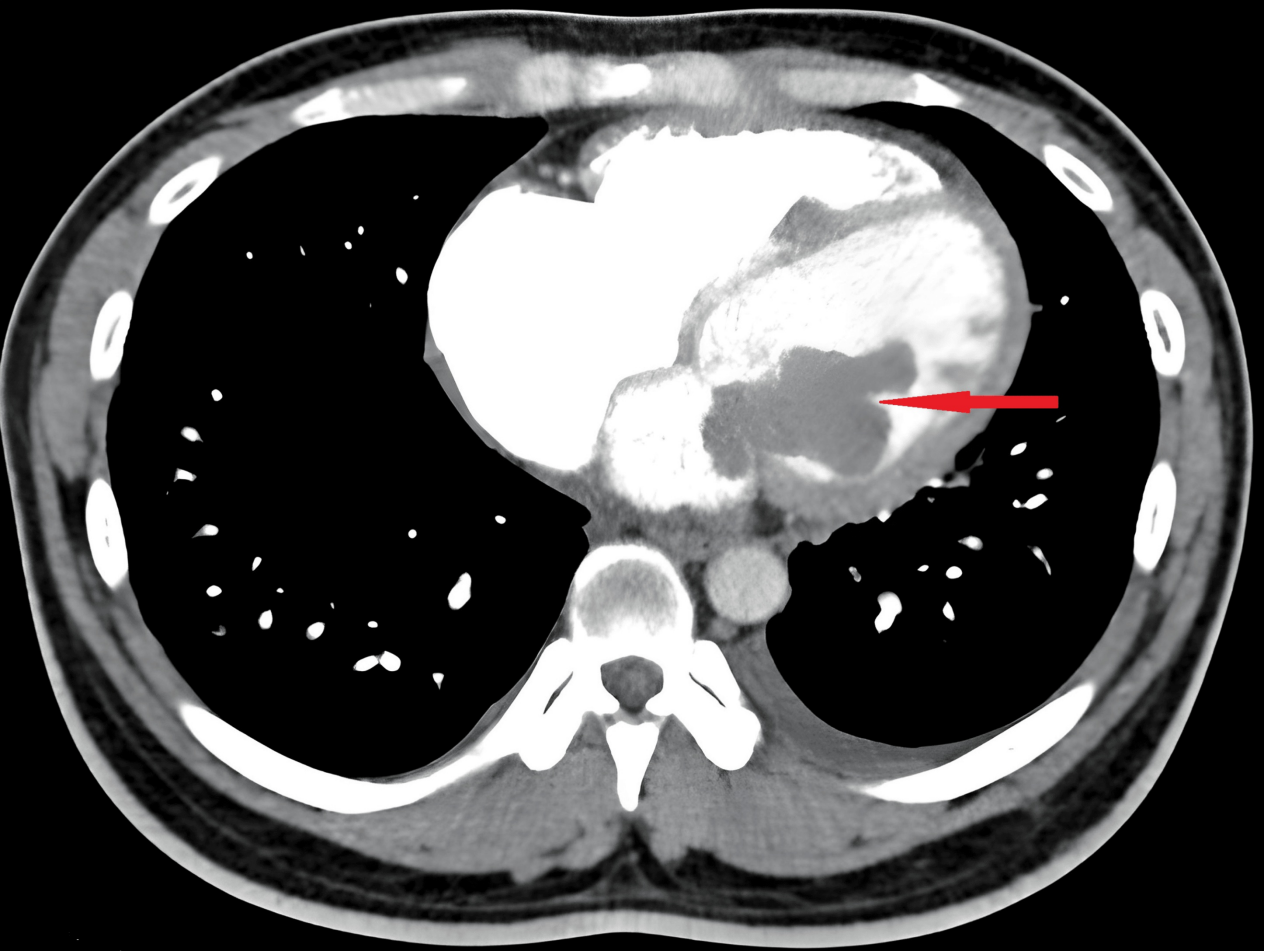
CT scan showing the presence of the left atrial mass prolapsing into the left ventricle (red arrow)
